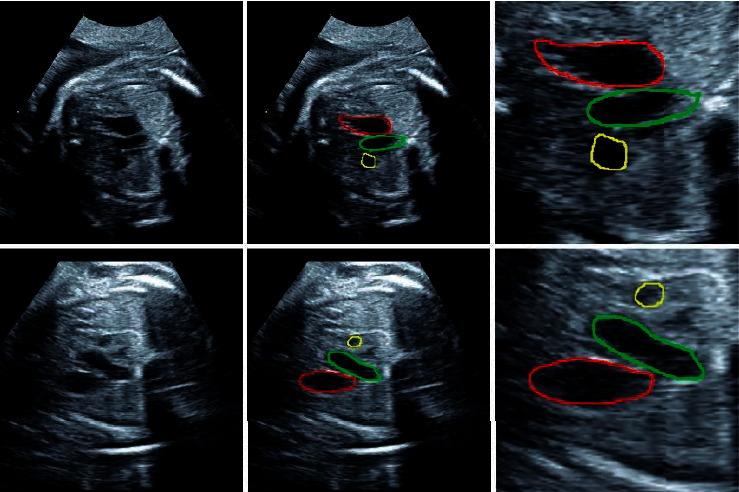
The 1st column shows original 3VT view of fetal heart ultrasound images. The 2nd column shows full-size labelled images, the red region is pulmonary artery (PA), the green region is aorta (AO), and the yellow region is superior vena cava (SVC). The 3rd column shows ROI-based cropped images, which are cropped from full-size images.
